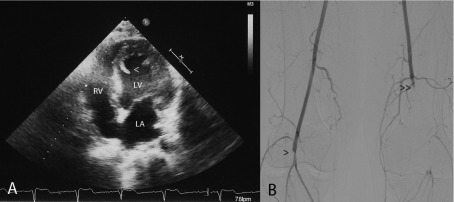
Echocardiogram and Lower Limbs Ateriography A: Apical four chamber echocardiographic view showing a thrombus, 23 mm long, attached to the ventricular septum in the left ventricular cavity (arrowhead). B: Lower limbs arteriography showing right popliteal artery (arrowhead) and left superficial femoral artery (double arrowhead) occlusion with a poor collateral circulation. LV: left ventricle, RA: right atrium, RV: right ventricle.
